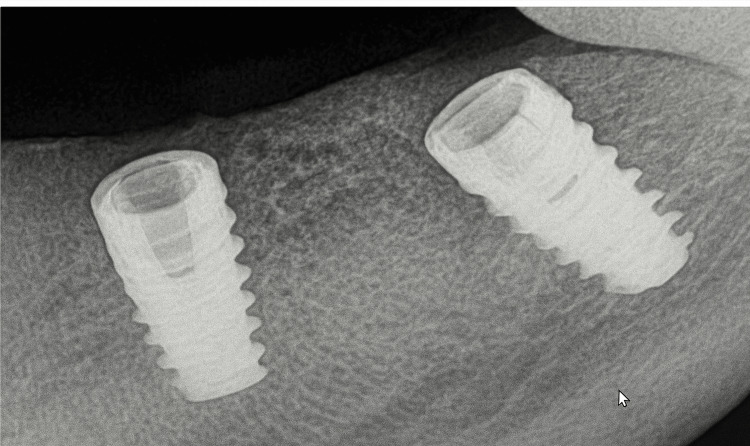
Intraoral periapical radiograph showing implants placed after ridge split procedure
